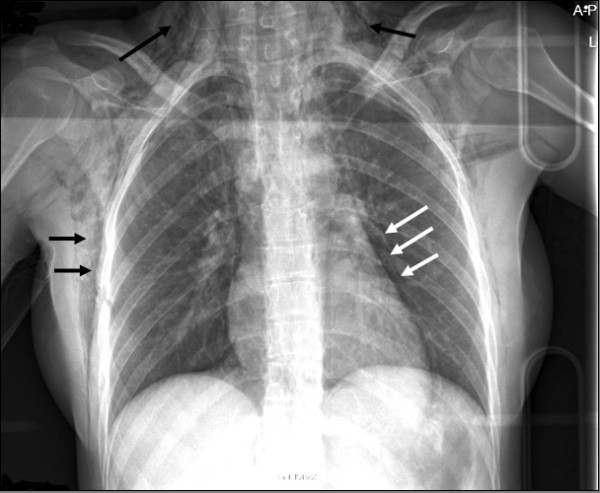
X-ray of chest on day of injury. Black arrows: subcutaneous emphysema. White arrows: pneumomediastinum
